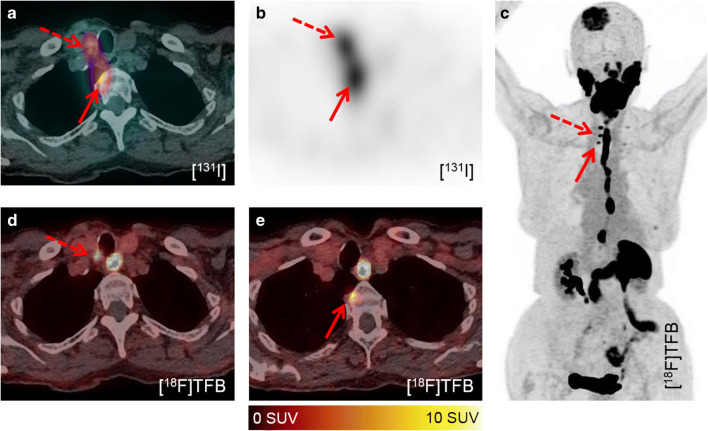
Superior image quality of [^18^F]TFB-PET-CT. In contrast to all other cases, patient #12 (92 years; TG, 5009 ng/ml; antiTG, 22.6 IU/ml; follicular DTC) received a therapeutic activity of [^131^I]iodine for imaging and therapy due to already known metastases. However, the delineation of local recurrence (dashed arrow) and a bone metastasis (arrow) is difficult in the SPECT-CT fusion (a). The transaxial SPECT reconstruction enables a better discrimination (b). However, [^18^F]TFB-PET-CT enabled superior delineation of metastases and local recurrence (c–e). Note additional bone metastases, e.g., skull
